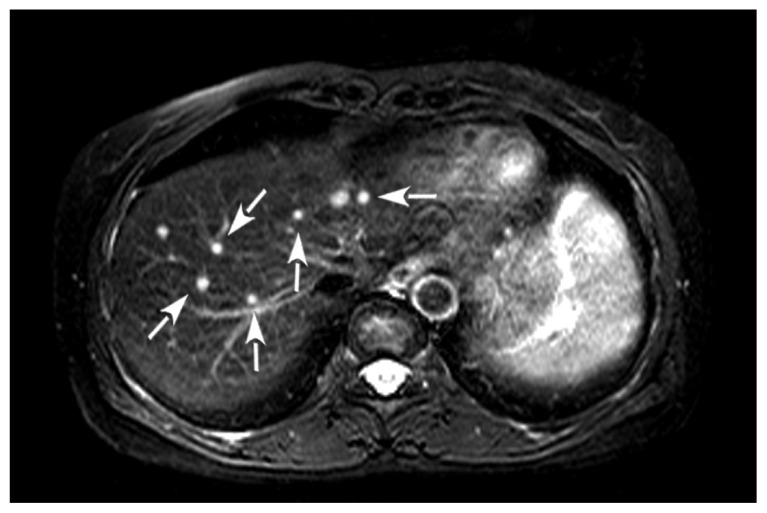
Horizontal abdominal MRI image in May 2006 shows multiple solid nodules in the right lobe of the liver (arrows indicated).
